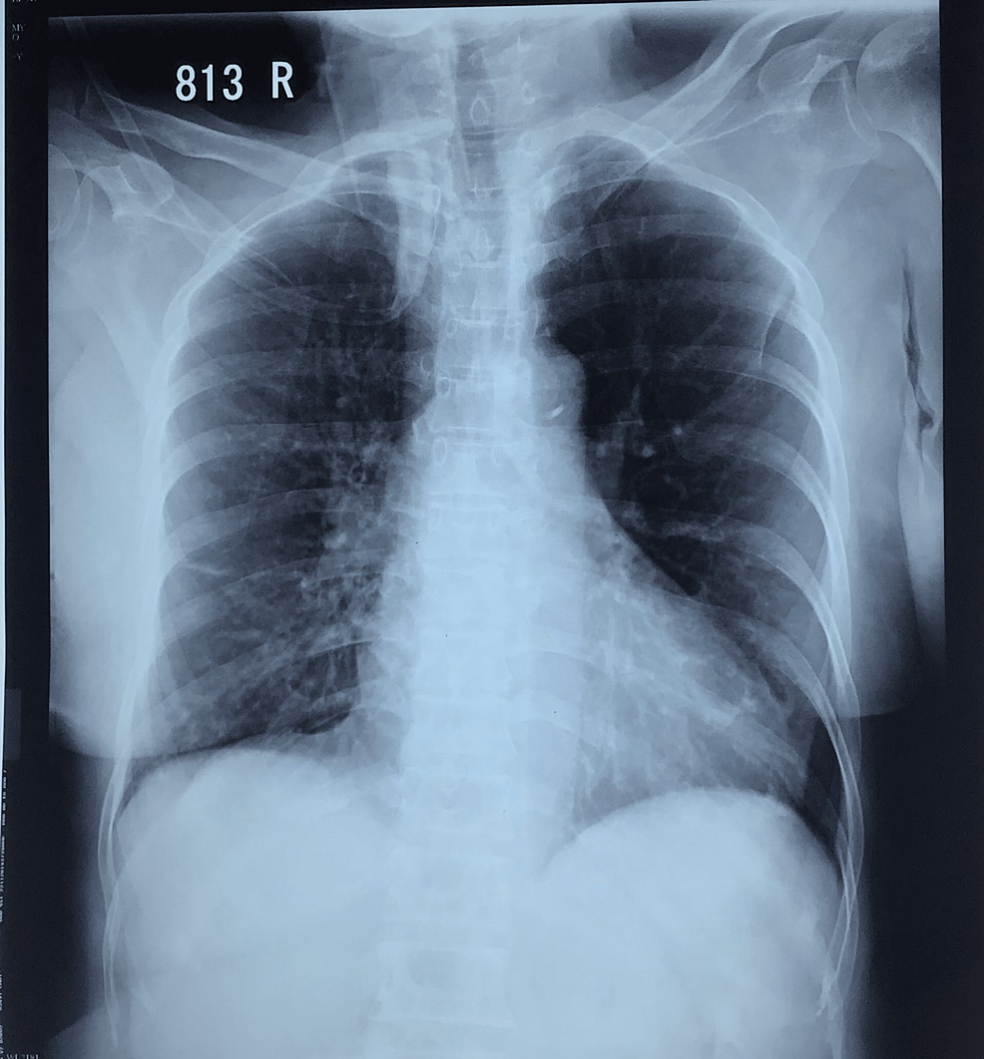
The chest X-ray showing early pulmonary edema.
